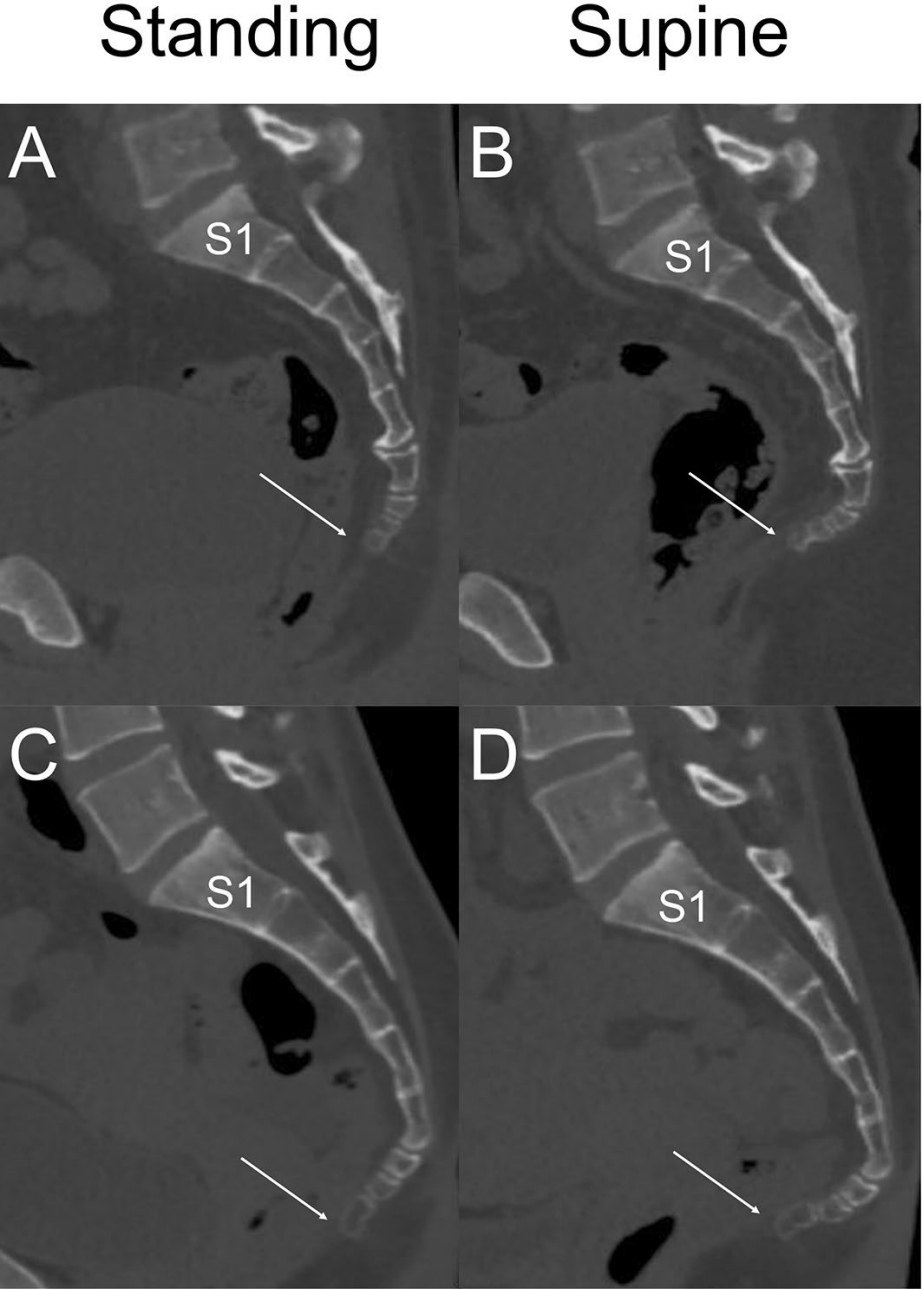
Computed tomography imaging of the coccyx in healthy volunteers. The coccyx of a 36-year-old man in the standing position (A) and the supine position (B), and the coccyx of a 47-year-old parous woman in the standing position (C) and supine position (D) in the mid-sagittal plane. As demonstrated, the coccyx becomes longer and straighter, with the tip of the coccyx (white arrow) moving backward and downward relative to the supine position.
